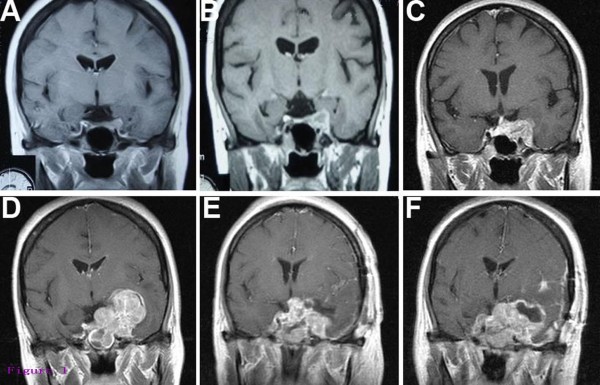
Coronal MRI scans of malignant myoepithelioma development in the present case. Initial T1-weighted post-contrast images taken at symptom onset were normal (A). Follow-up images 1 month later depicted a new, left cavernous sinus mass with heterogeneous signal intensity (B). Preoperative images indicated the tumor mass significantly increased (C). Post-biopsy images displayed more progressive tumor development, since the tumor filled the left cavernous sinus and extended into the ipsilateral middle fossa (D). Postoperative images presented a large residue with successive tumor growth (E, F).
